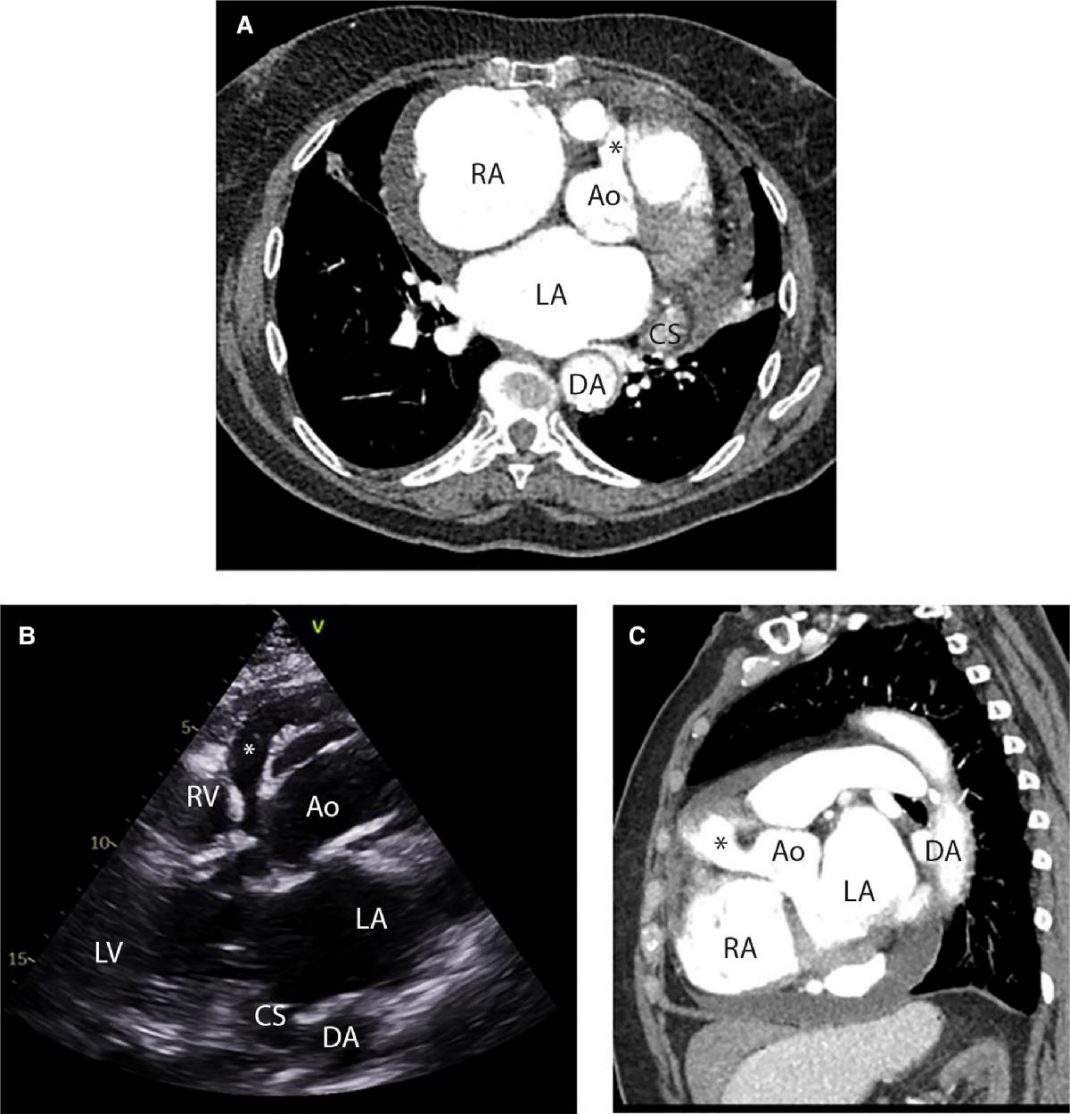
Pre-operative images demonstrating the origin of the aneurysmal right coronary artery arising from the right coronary sinus (marked with asterisk) and its relationship to adjacent structures. (*A*) Axial oblique CT, (*B*) parasternal long axis view from TTE, (*C*) sagittal oblique CT. RA, right atrium; LA, left atrium; Ao, aortic root; LV, left ventricle; RV, right ventricle; DA, descending aorta; CS, coronary sinus.
